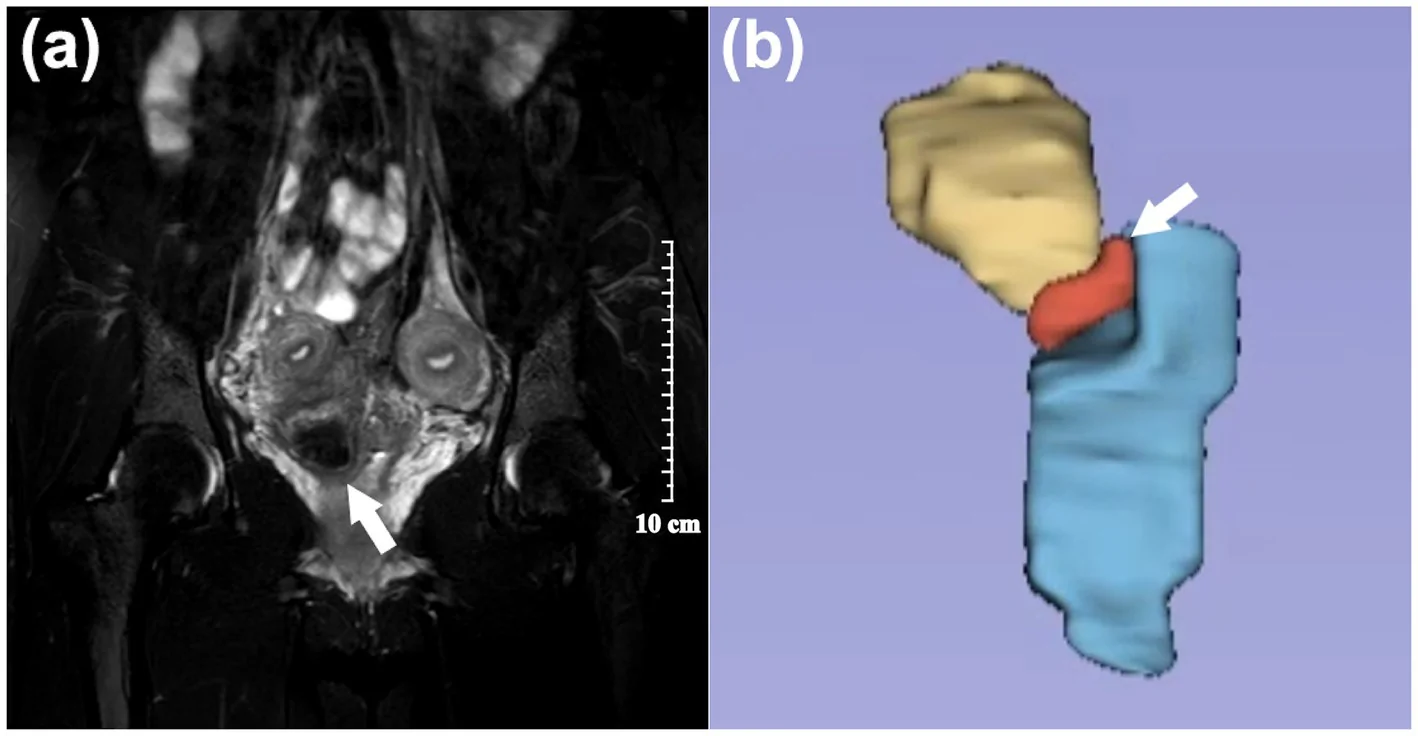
Preoperative MRI (a) Sagittal T2-weighted MRI showing uterus didelphys (U), obstructed right hemivagina with hematocolpos (H), and the oblique vaginal septum (arrow). The septum measured 11 mm in thickness and 13 mm in length. (b) Three-dimensional reconstruction demonstrating two separate uterine cervices (arrows) and left renal agenesis.
